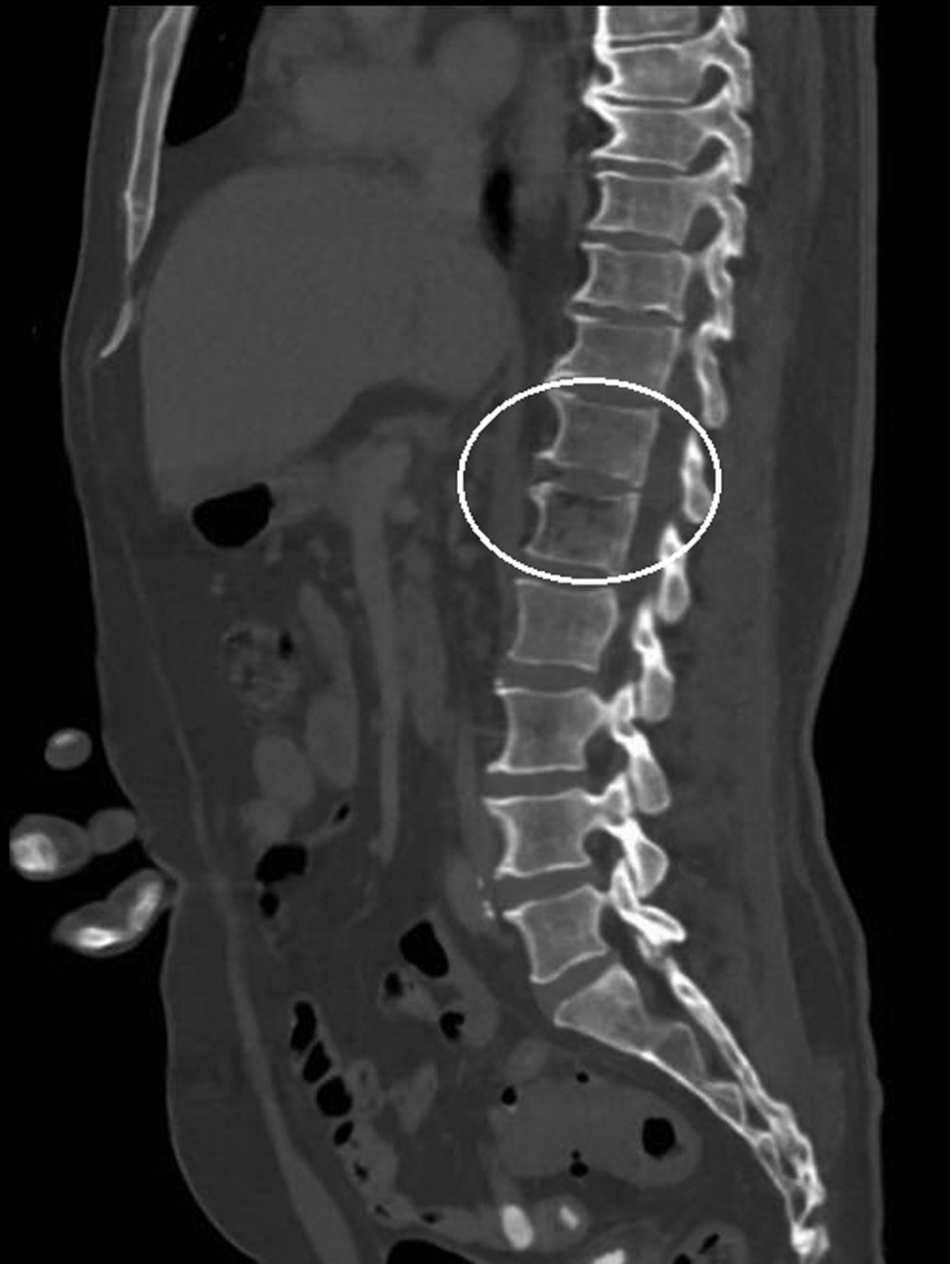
A sagittal bone view of computed tomography of the abdomen and pelvis revealing gas within the twelfth thoracic and first lumbar vertebral body (circle) suspicious for osteomyelitis.
